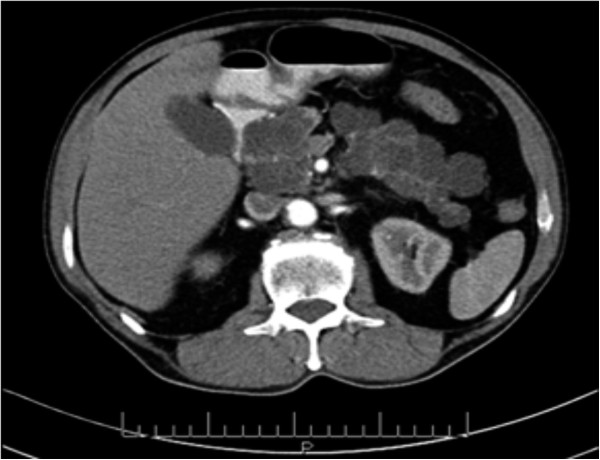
Contrast enhanced computed tomography showing multiple, variably sized cystic lesions with multiple calcific foci involving the whole pancreas.
